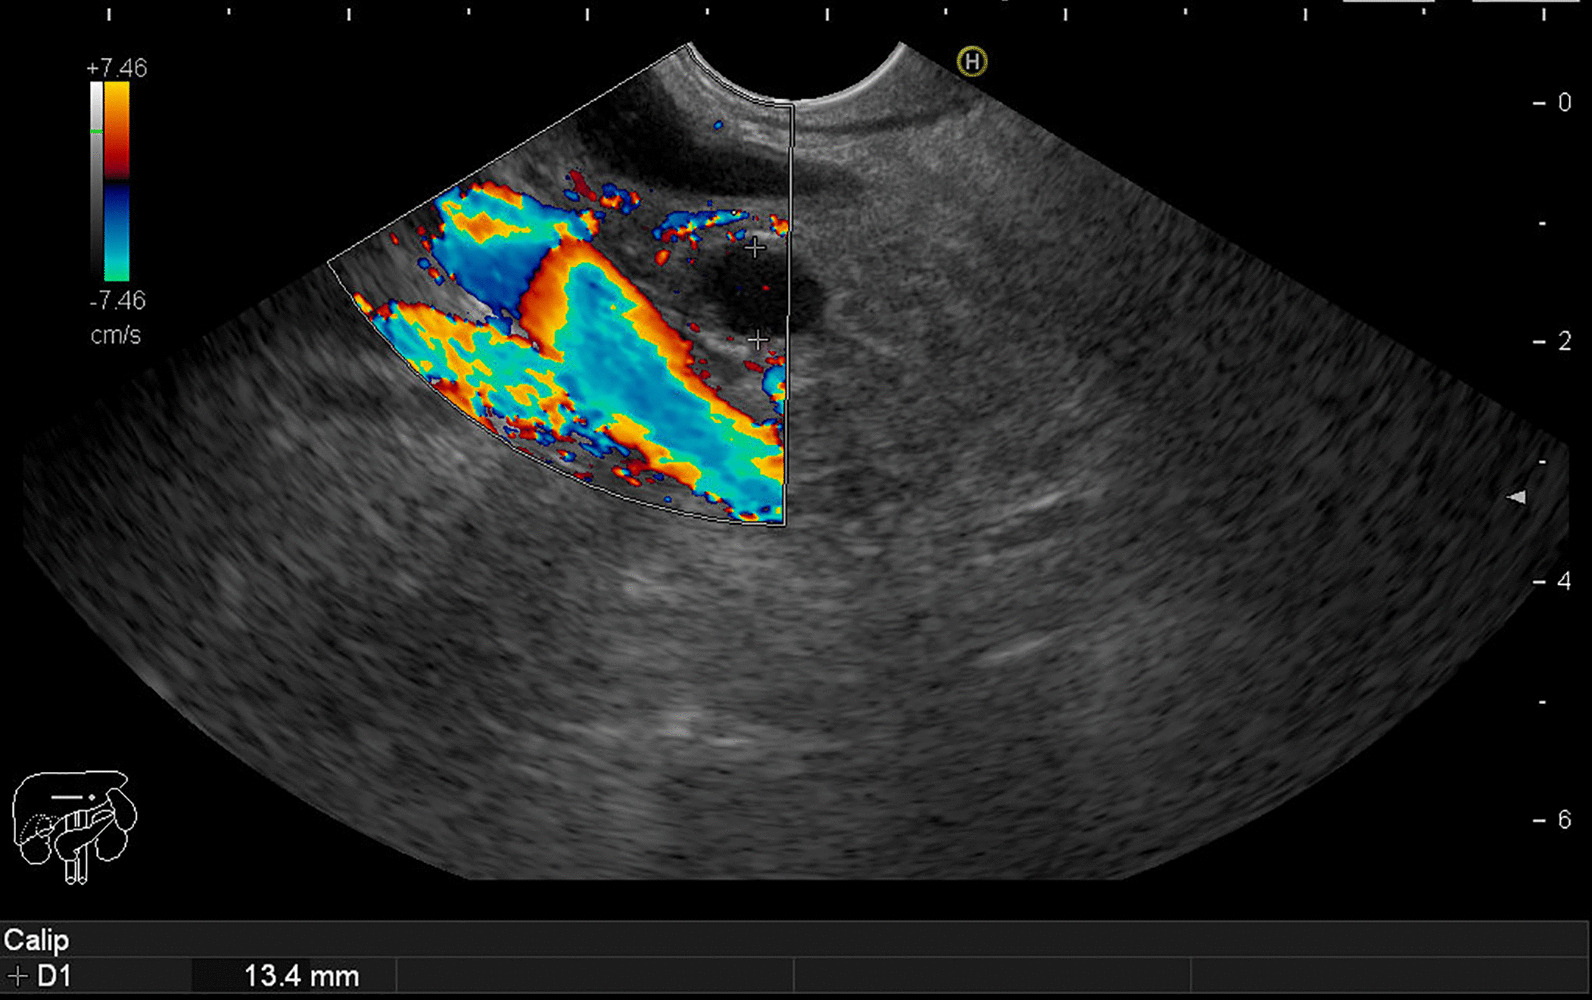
Endoscopic ultrasound showing a dilated common bile duct
